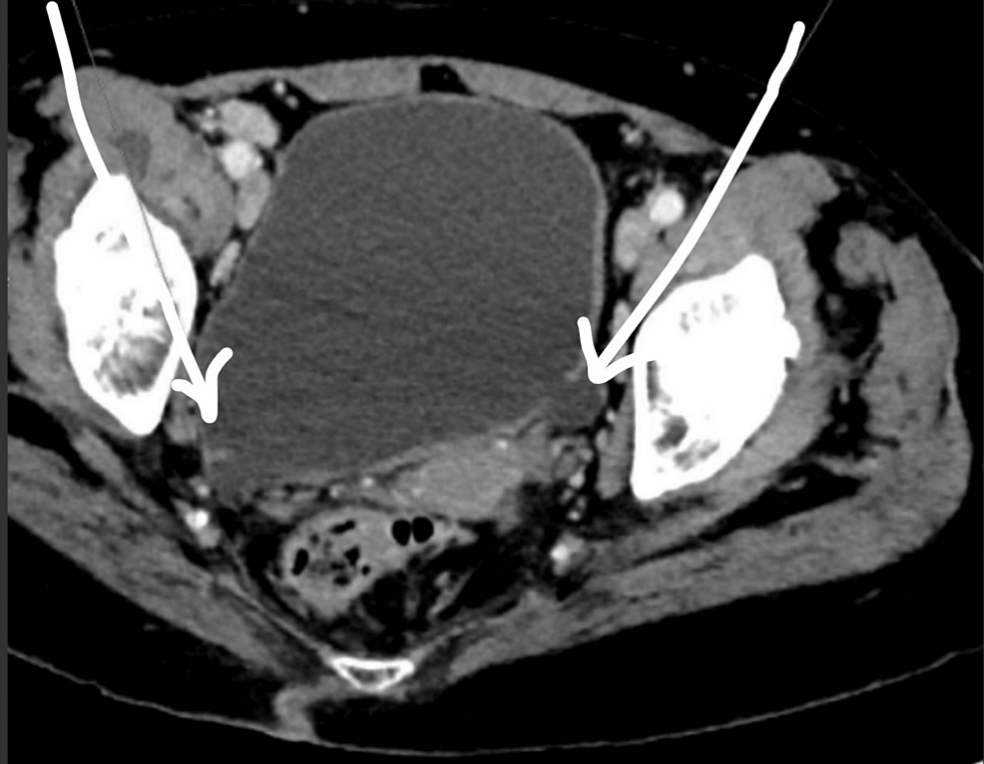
A CT abdomen and pelvis showing left and right multiple bladder diverticula with white arrows inserted demonstrating these multiple diverticula.
